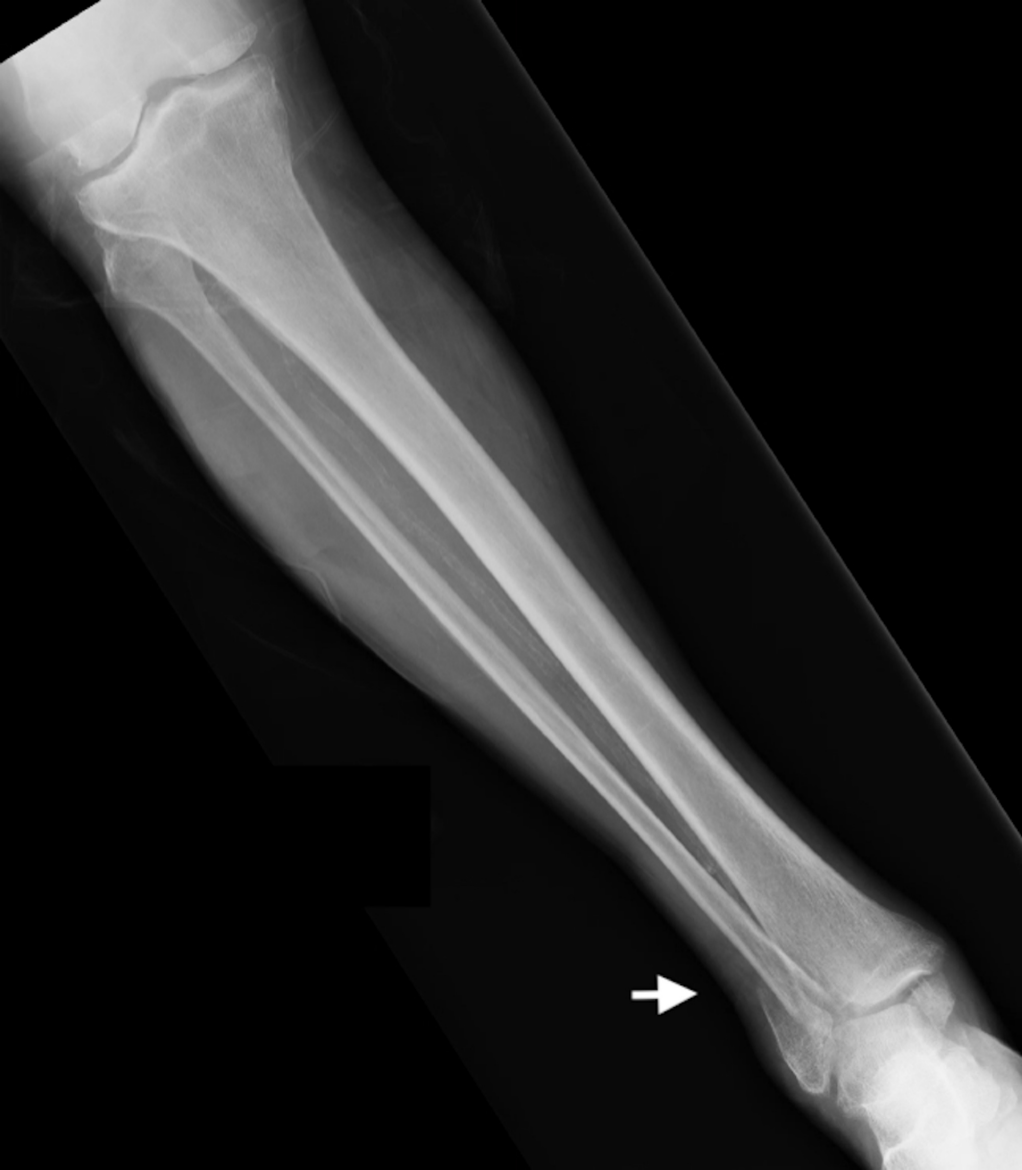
X-ray of right leg showing distal fibular fracture (arrow)
